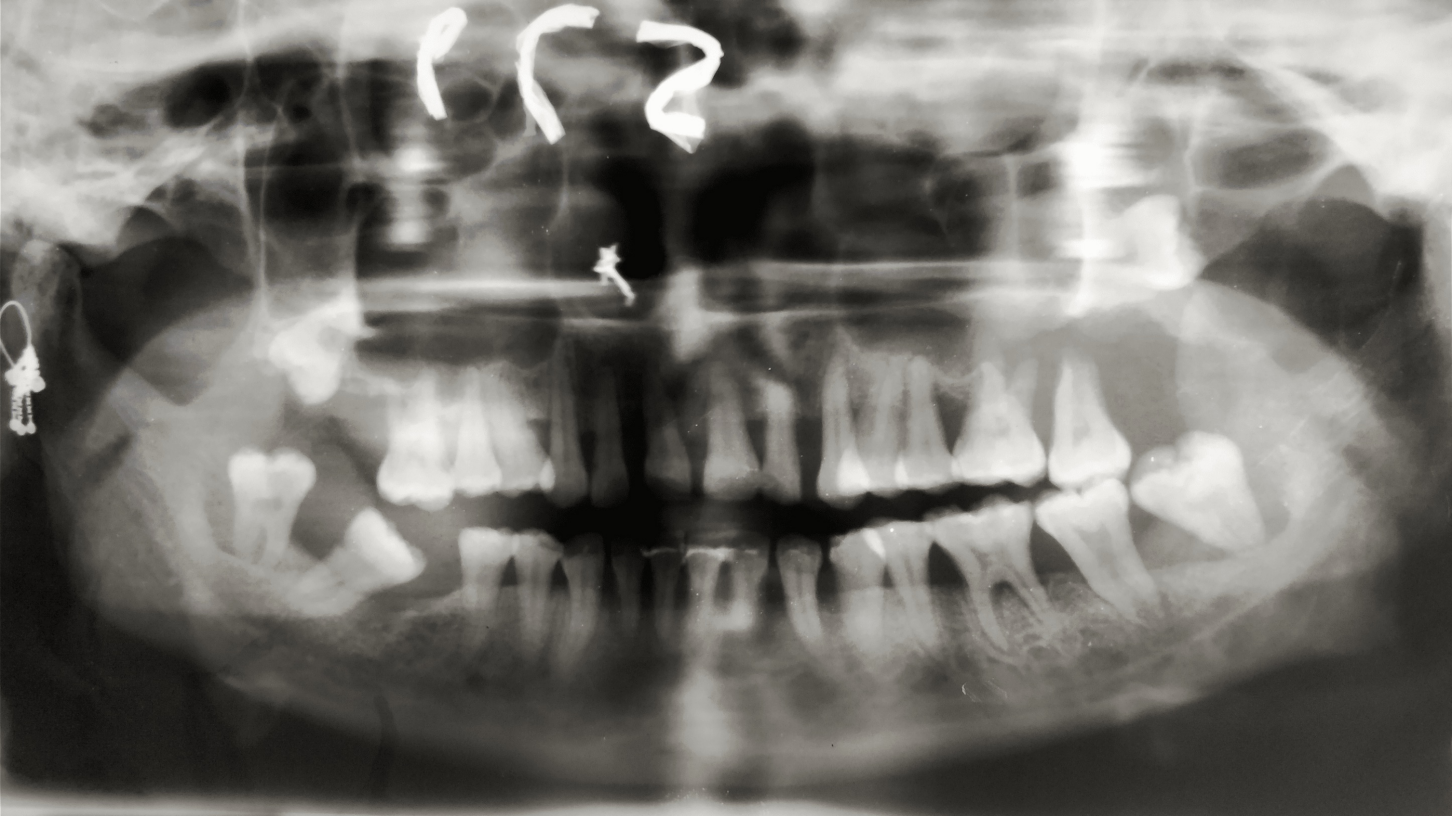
OPG showing generalized bone resorption and root resorption with tooth no. 16. The floor of the maxillary sinus was not traceable, with impacted teeth 18 and 28, with root portions extending into the maxillary sinus. A generalized interdental bone loss was observed in the mandible, with severe bone loss in teeth 37, 38, 47, and 48, as well as displaced teeth 37, 38, and 48 OPG = Orthopantomogram
